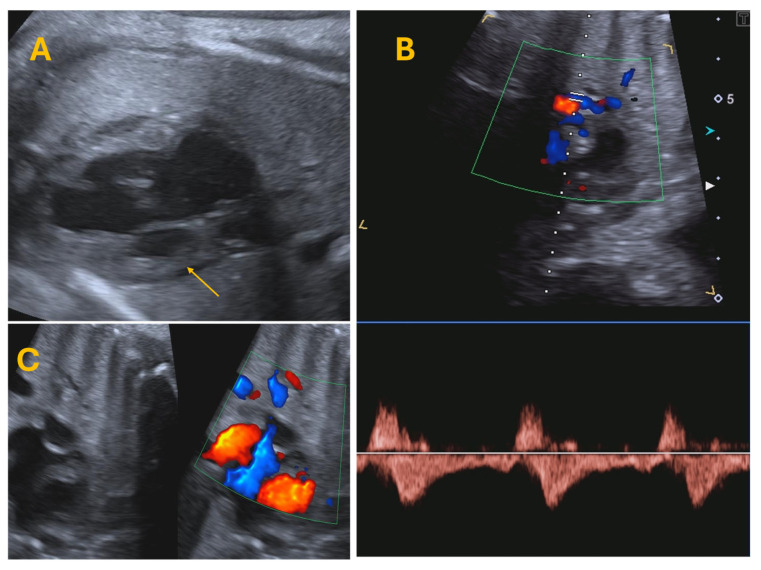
(A) four chamber view showing an example of Hypoplastic Left Heart Syndrome (arrow). (B) PV PW Doppler analysis, showing a reversed A wave. (C) PFO investigation, showing a thick PFO with left-right shunt.
